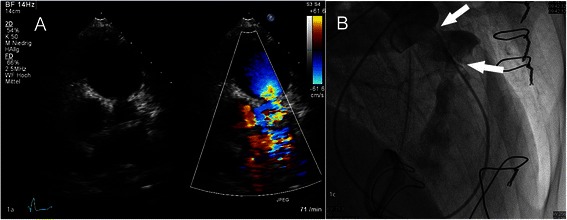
Transthoracic echocardiography and angiography of patient no. 1. A: Transthoracic echocardiography of the degenerated pulmonal valve prosthesis in doppler color flow with signs of stenosis. B: Angiography with stenosis of the proximal and distal anastomoses (see markers) of the bioprosthesis.
